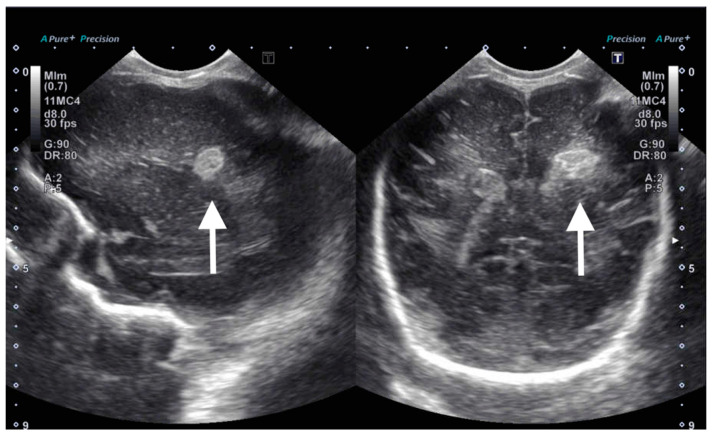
Sagittal (left) and coronal (right) views of cranial ultrasound from day of life 5 showing focal echogenicity on the left side of the posterior frontal lobe white matter (arrows) with no intraventricular hemorrhage or ventriculomegaly.
